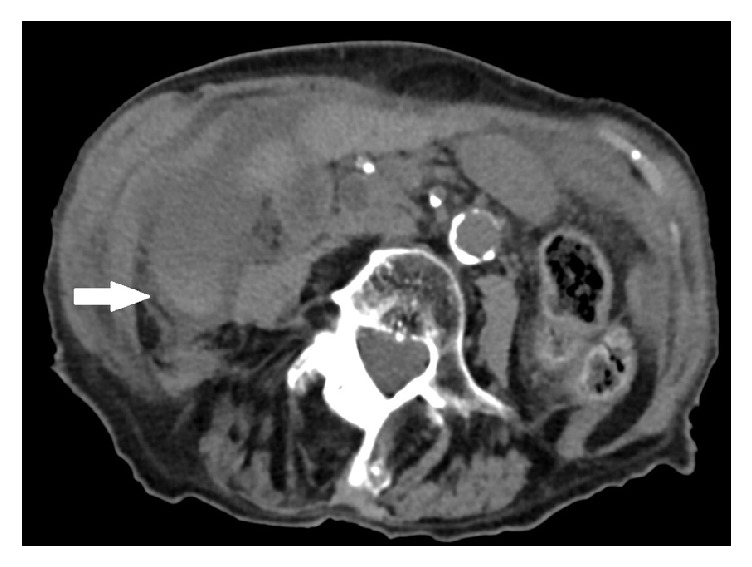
Abdominal unenhanced CT scan: high attenuation area, suggesting an intraluminal hemorrhage.
